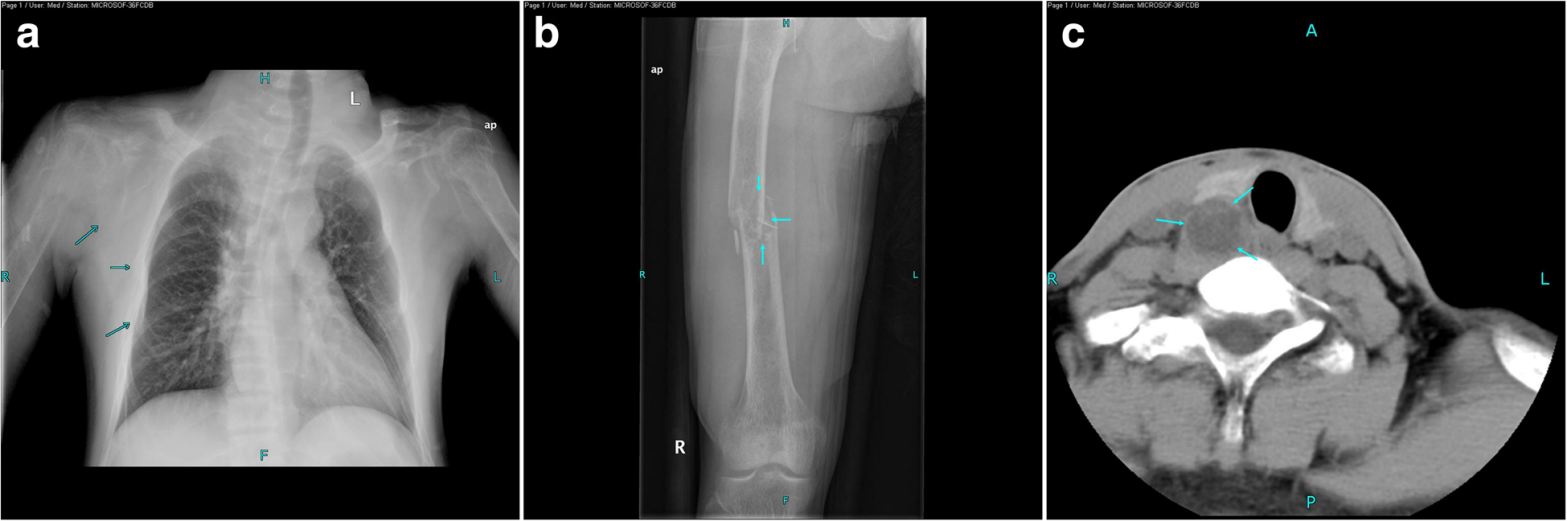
(b) Radiographs of a pathological fracture of the right femur. (a) Chest radiograph showing extensive osteolysis of the thoracic bones and several old fractures on the right scapula and ribs (arrow). (c) CT scan of the neck (axial view) showing a 3.5 × 2.5 × 2.0cm nodular mass on the right parathyroid
